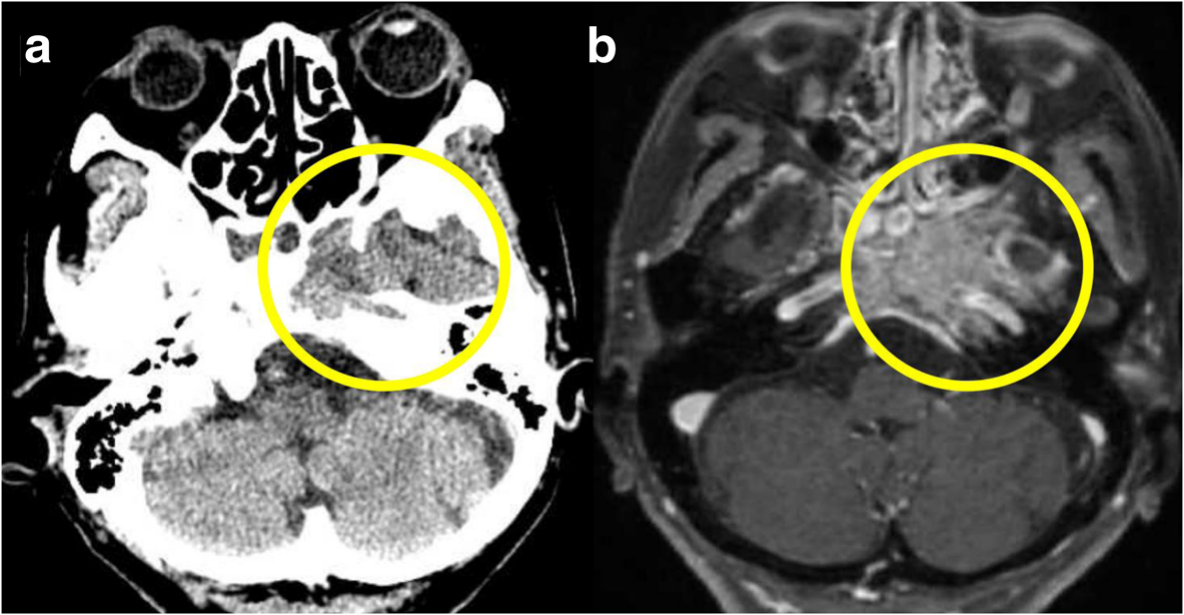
a Head CT shows bone destruction at the left sphenoid sinus. b Gadolinium-enhanced MRI (T1WI) of the brain shows a contrast-enhanced tumor at the left sphenoid sinus
